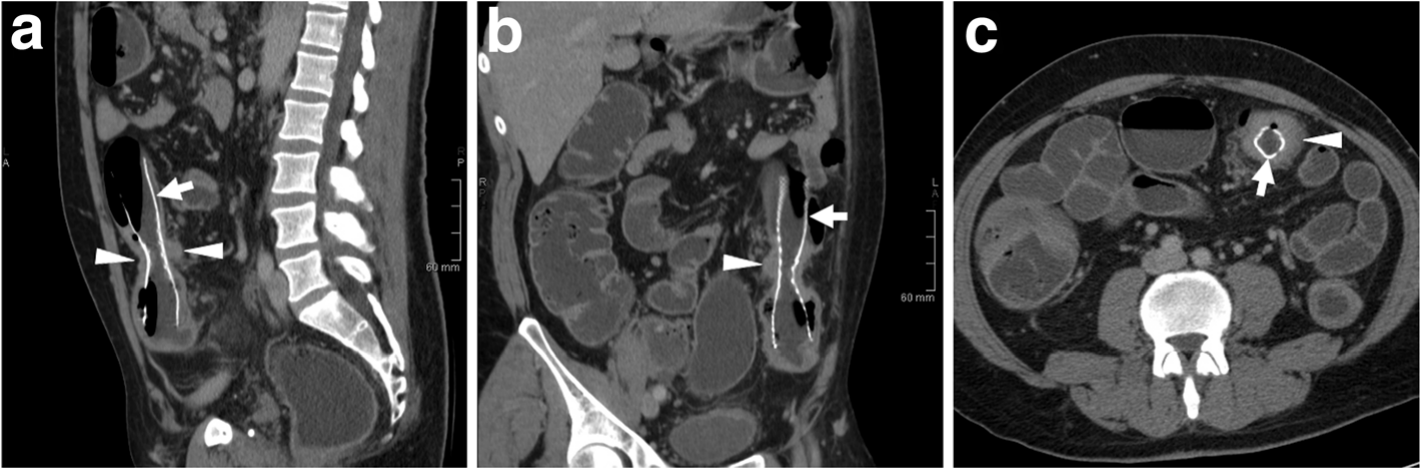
Comprehensive staging of SEMS-treated CRC using water enema CT. Multiplanar contrast-enhanced images (a–c) show sufficiently expanded SEMS (thick arrows) at a stricturing tumour of the descending colon, seen as circumferential enhancing mural thickening (arrowheads). The upstream large bowel is adequately distended by water and does not show additional suspicious changes
